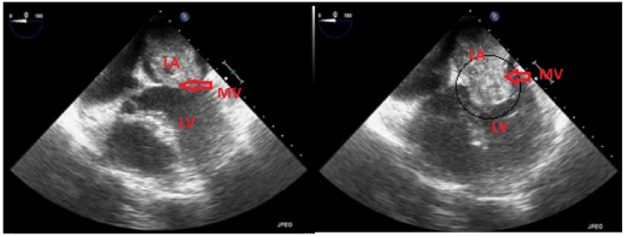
Transesophageal echocardiogram of the large obstructing left atrial myxoma. The dimensions of the LA myxoma were 6.1 cm × 4.5 cm and occupied most of the volume of the LA and caused severe obstructive stenosis across the mitral valve. The patient was also found to have severe pulmonary hypertension (LA, left atrium; LV, left ventricle; MV, mitral valve).
